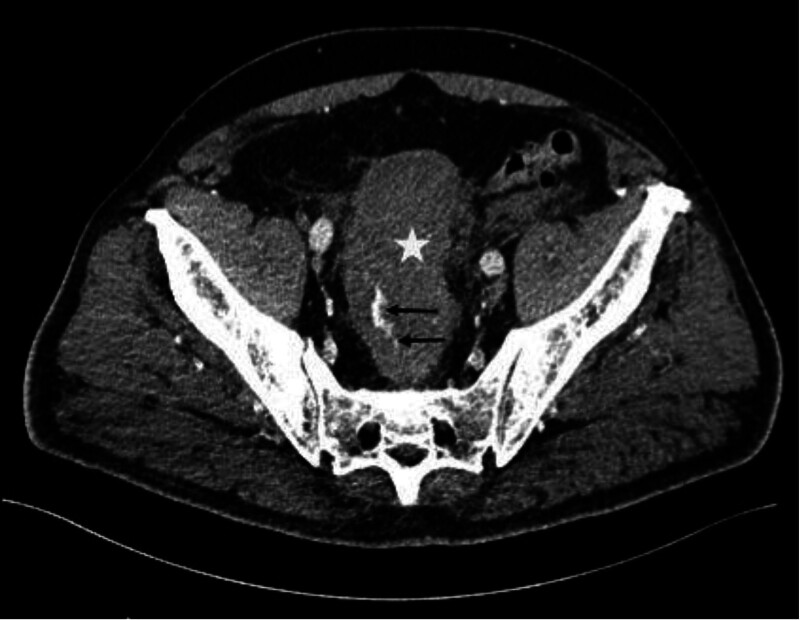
Active bleeding (black arrow) into the lumen of the hematoma (white star) seen on computed tomography scan.
